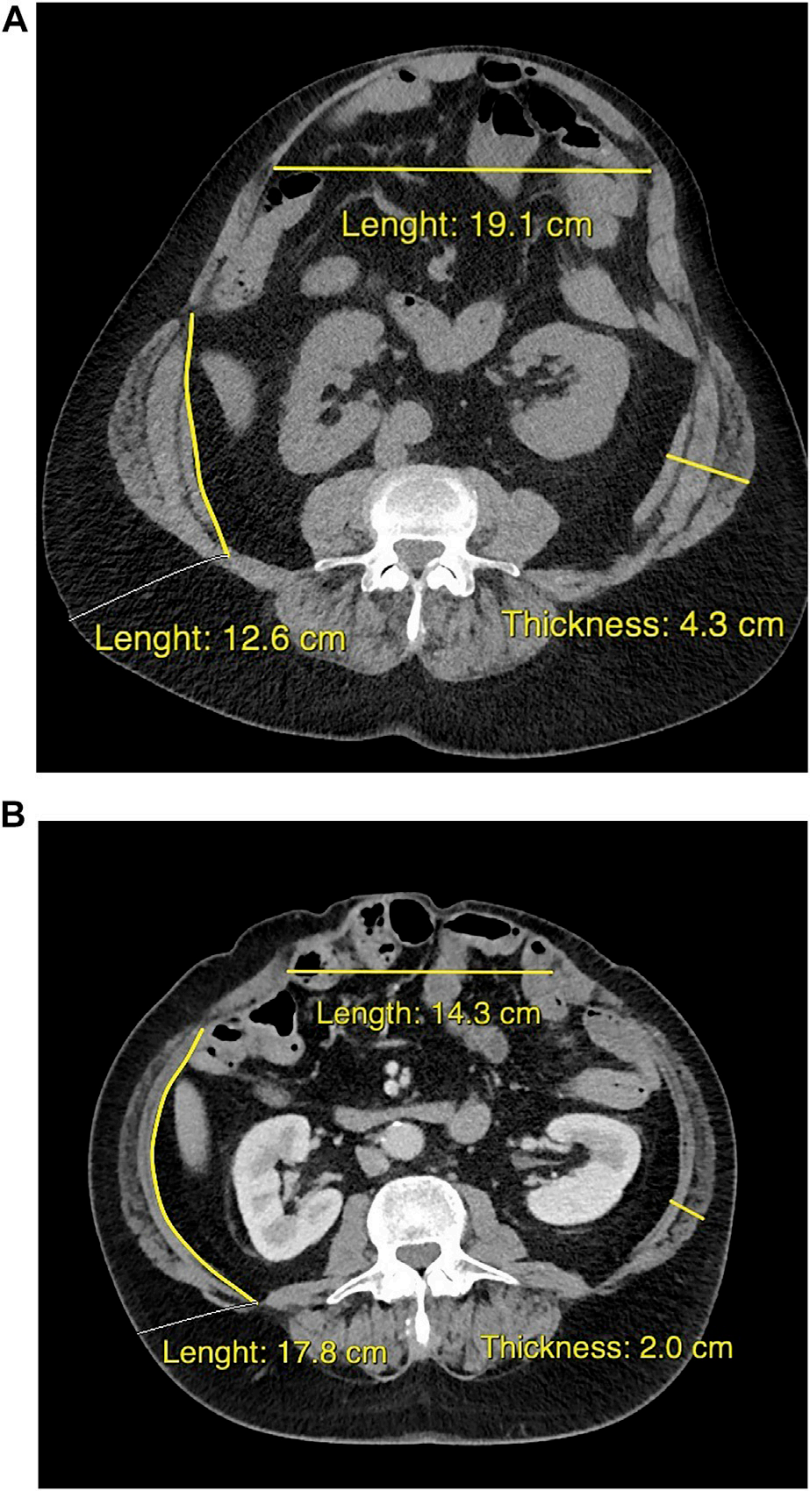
Cross-sectional CT images from the same patient at approximately the same transverse level, when discussed for surgery at the outpatient clinic (A), and about 5 weeks after bilateral intramuscular injection with botulinum toxin (B). The pretreatment flattened and elongated the lateral abdominal wall muscles, and reduced the hernia diameter.
